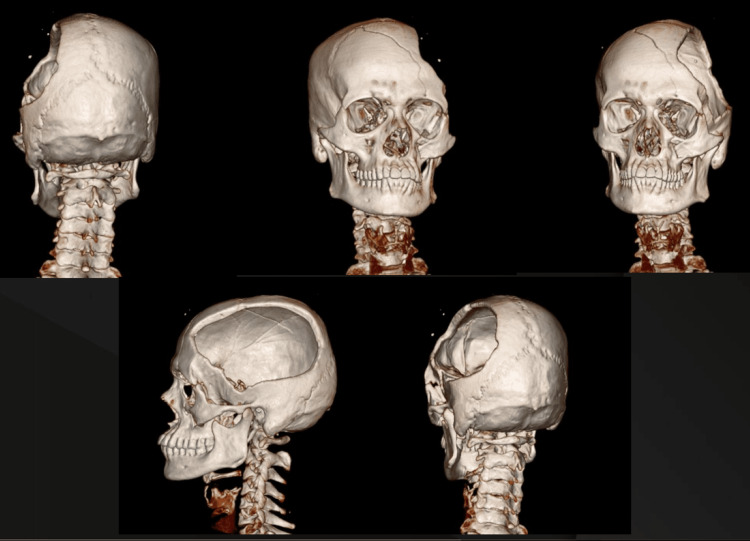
Reconstructed 3D images from CT scan
